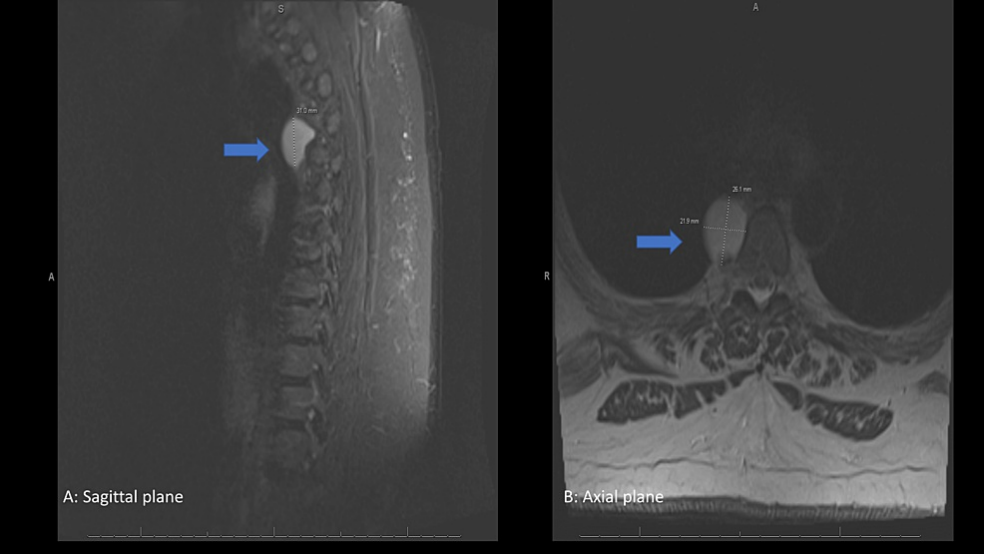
MRI of the thoracic spine. MRI of the thoracic spine showing the cyst (blue arrow) in (A) sagittal and (B) axial plane.
